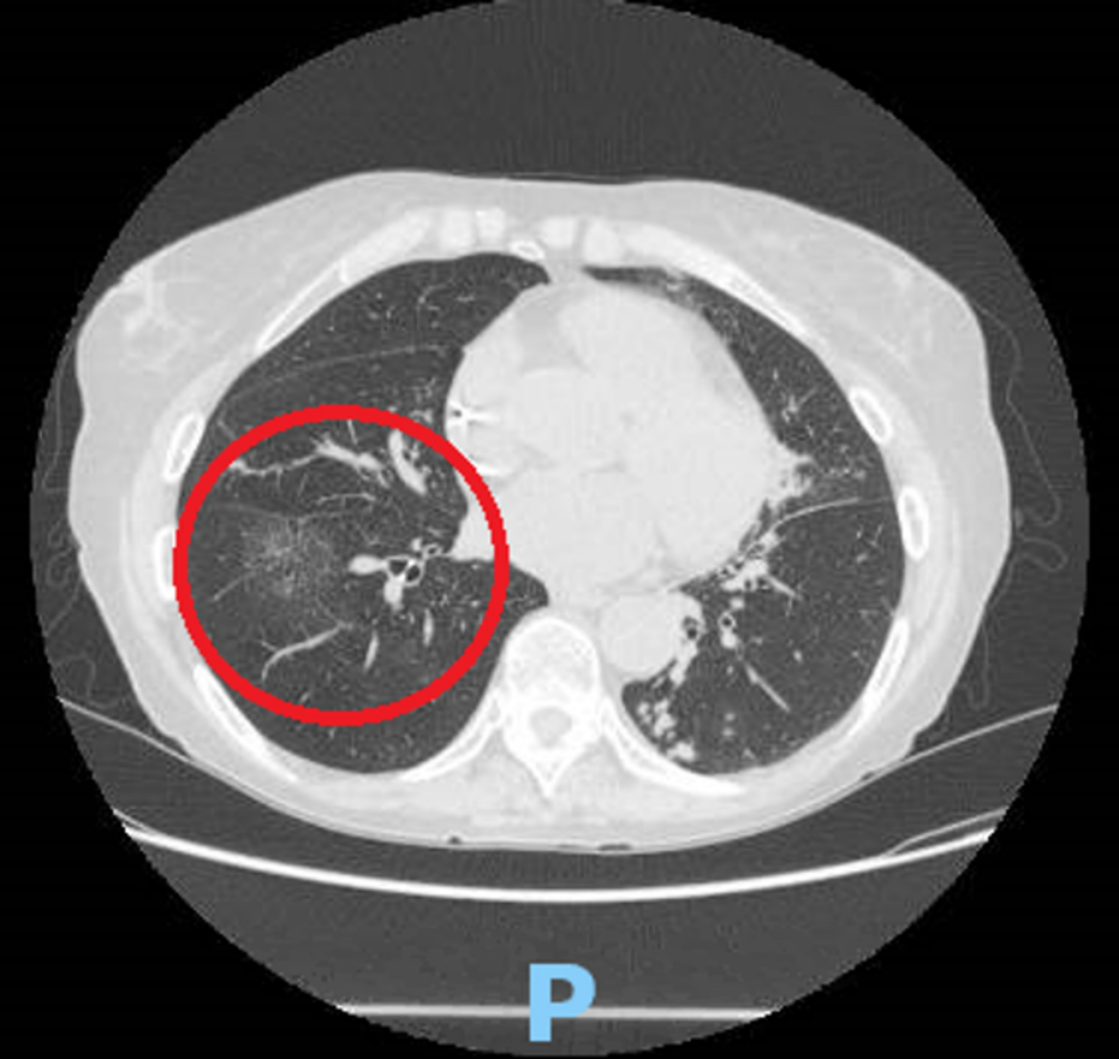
Non-contrast computed tomography of the chest, axial view, showing a new ground-glass opacity of the right lower lung field (red circle).
